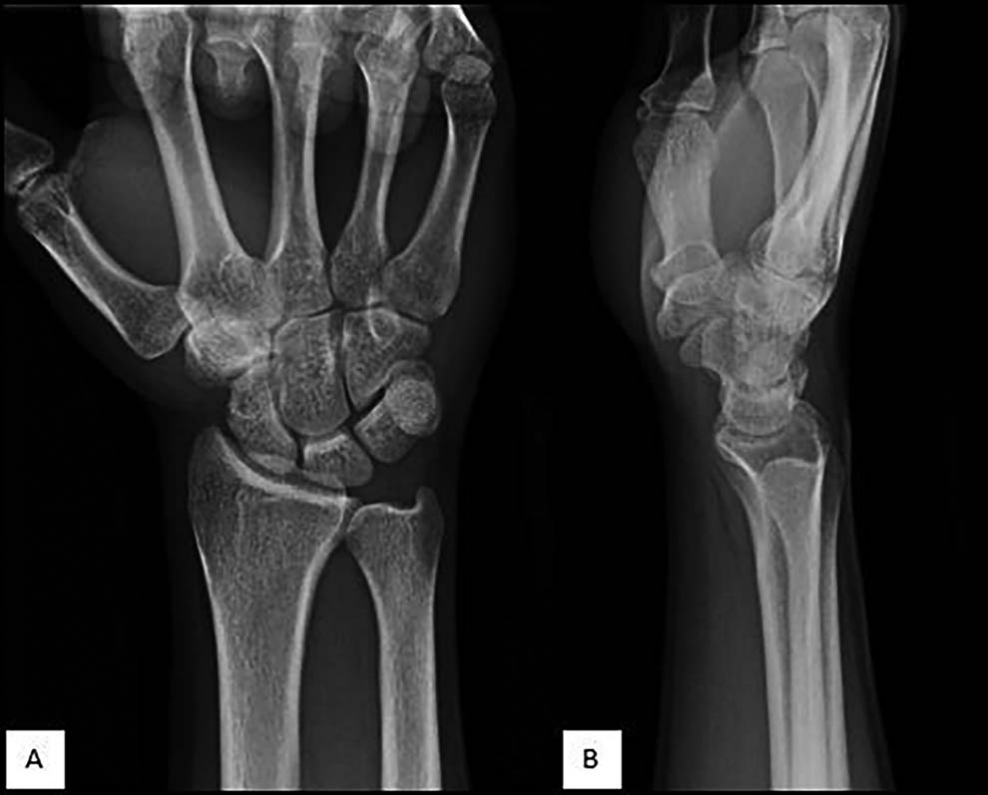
Unremarkable (A) posteroanterior and (B) lateral radiographs of the right wrist show no evidence of acute fracture.
